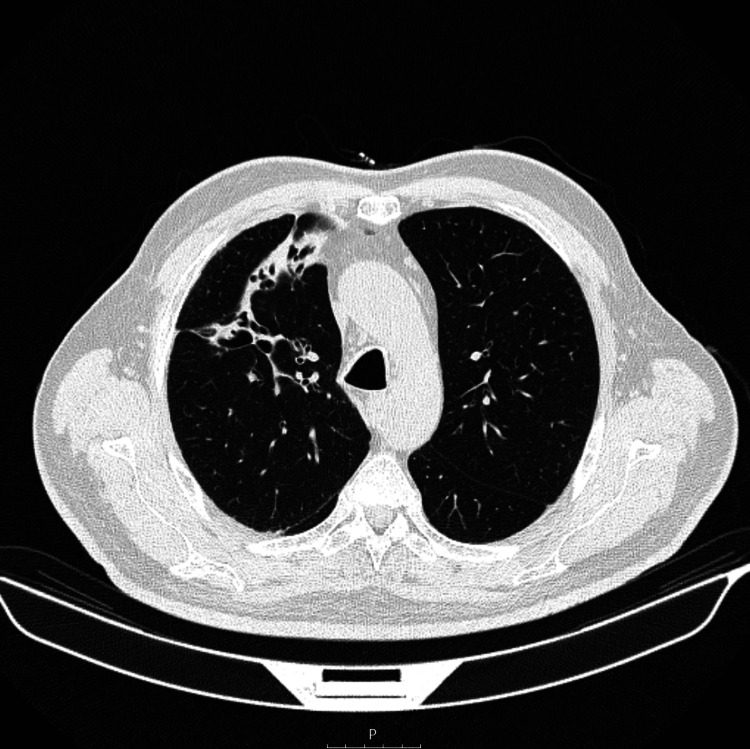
Chest computer tomography after six months of treatment Chest computed tomography reevaluation at the end of the treatment with complete imaging remission, although with sequelae bronchiectasis.
